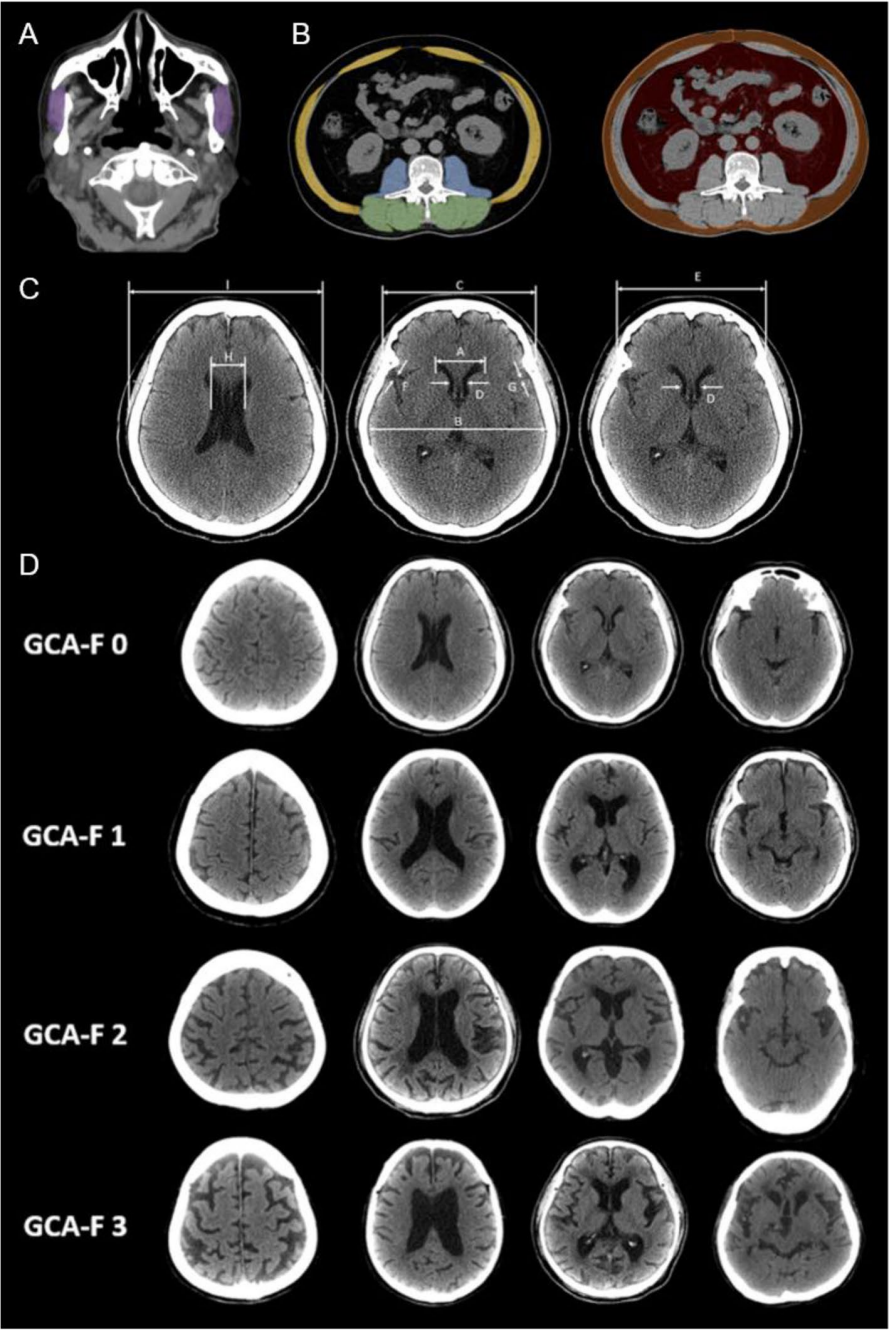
CT image analysis of body composition and brain atrophy. A. Head CT analysis of masseter muscle area. (Purple area: masseter muscle). B. Abdominal CT analysis of skeletal muscle area at third lumbar vertebral level. (Yellow area: abdominal wall muscle; Blue area: psoas muscle; Green area: paraspinal muscle; Orange area: subcutaneous adipose tissue; Red area: visceral adipose tissue). C. Measurements of brain atrophy parameters: A, B, C, D, E, F, G, H, I, in head CT images. Evans index (EI): ratio of maximum width of the frontal horns of the lateral ventricles (A) to the maximal internal diameter of the skull; Frontal horn index (FHI): ratio of the greatest external diameter of the frontal bone (C) to the greatest distance between the frontal horns at the same line (A); Bicaudate ratio (BCR): ratio of the maximum caudate nuclei distance (D) to distance between inner tables of skull at the same line (E); (B) at the same level; Sylvian fissure ratio (SFR): sum of bilateral width of the insular cisterns (in mm) (F)(G); Schiersmann index: ratio of the lateral ventricles greatest distance at the level of cella media (H) to distance between outer tables of skull (I) at the same line; Huckman number: sum of maximum width of the frontal horns of the lateral ventricles (in mm) (A) and the maximum caudate nuclei distance (in mm) (D). D. Examples of frontal cortical atrophy (F-GCA) scale. GCA 0: normal sulci and ventricle; GCA 1: slight widening of sulci with mild ventricular enlargement; GCA 2: gyral volume loss with moderate ventricular enlargement; GCA 3: pronounced widening of sulci with severe volume loss (knife blade atrophy) and severe ventricular enlargement
